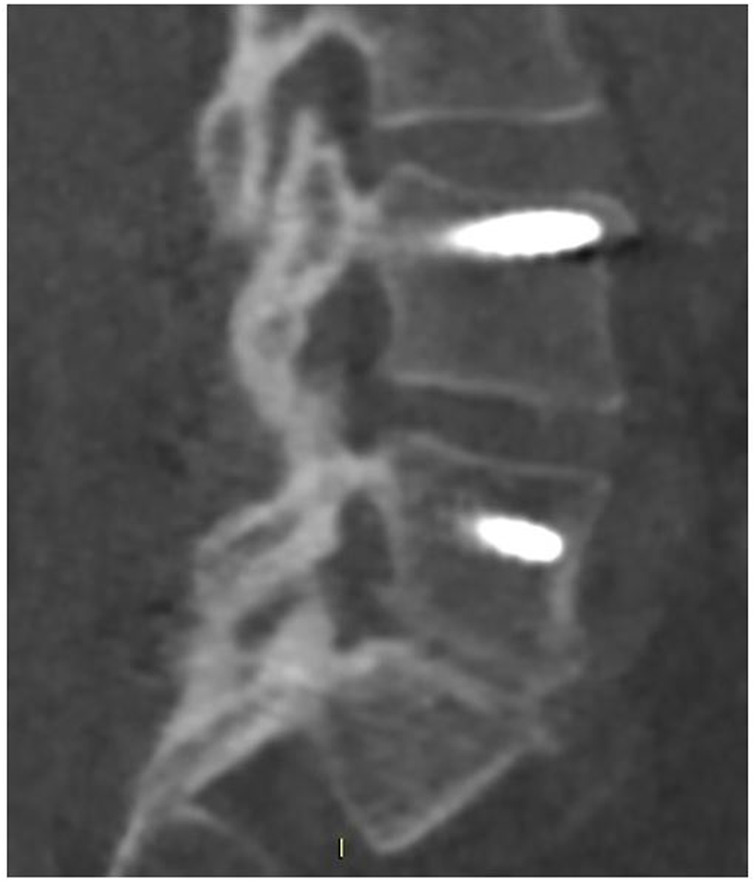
CT scan performed at 18-month follow-up, showing radiographic fusion at levels L4–L5 and L5–S1 where SintLife was used.
